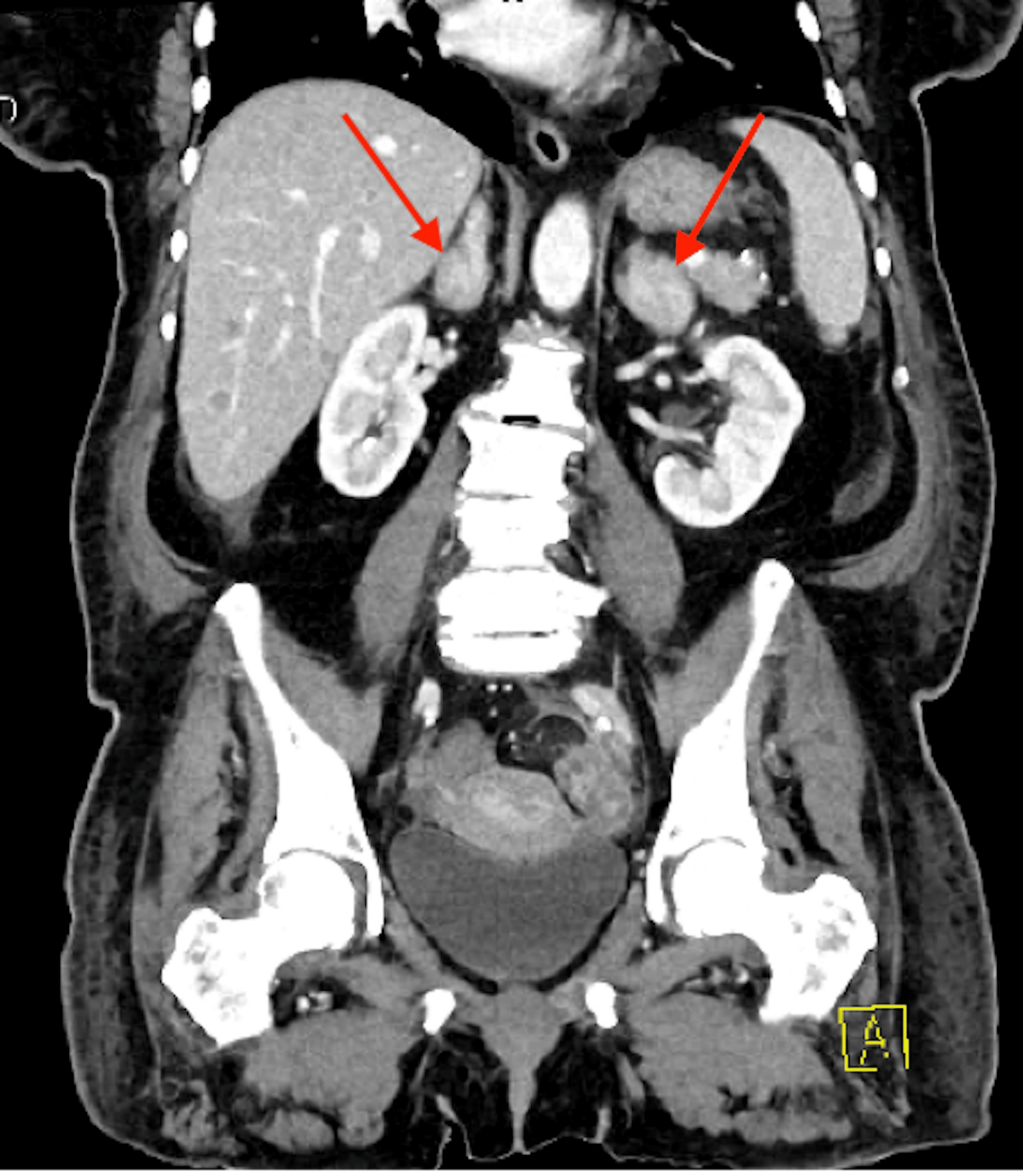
CT abdomen and pelvis with contrast displaying bilateral adrenal hyperplasia with multiple nodules (red arrows)
